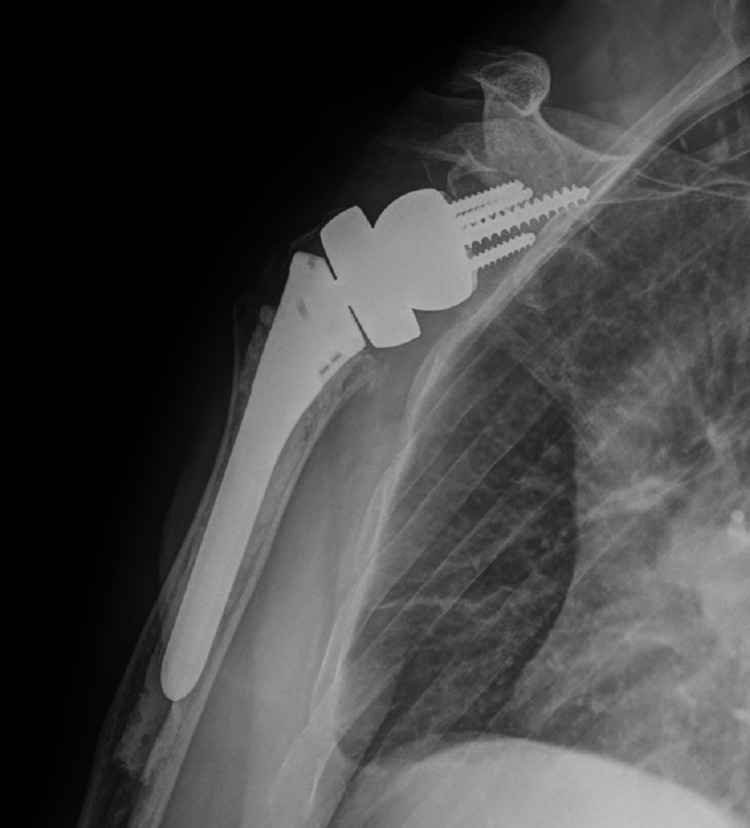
AP postoperative radiograph of the right shoulder at 12 weeks demonstrates a reverse total shoulder arthroplasty (rTSA) in satisfactory alignment without signs of loosening or failure.
